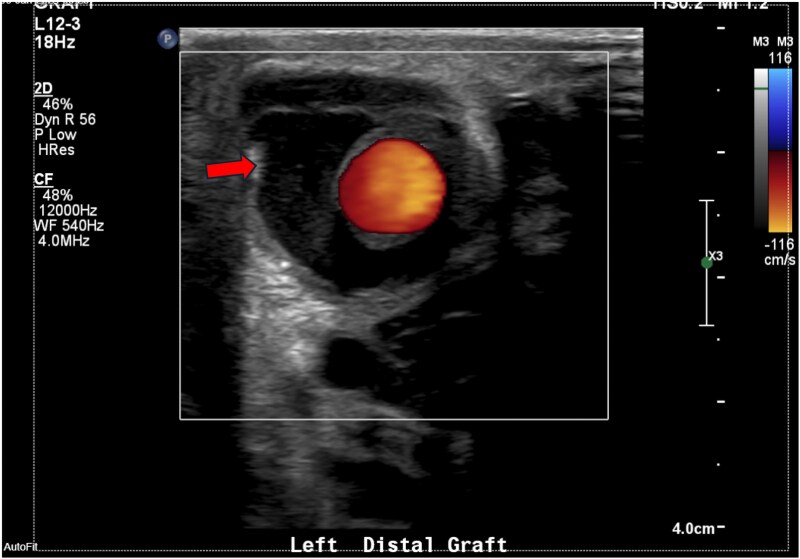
Ultrasonography of the graft site showing peri-graft fluid, ∼1.7 × 2.6 cm.
